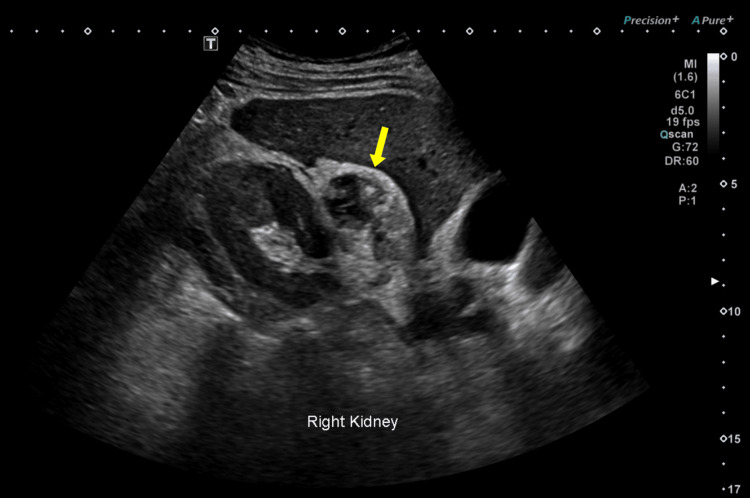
Retrospective ultrasound review revealing bowel wall signature within the suprarenal lesion (yellow arrow), supporting the diagnosis of a duodenal diverticulum.
